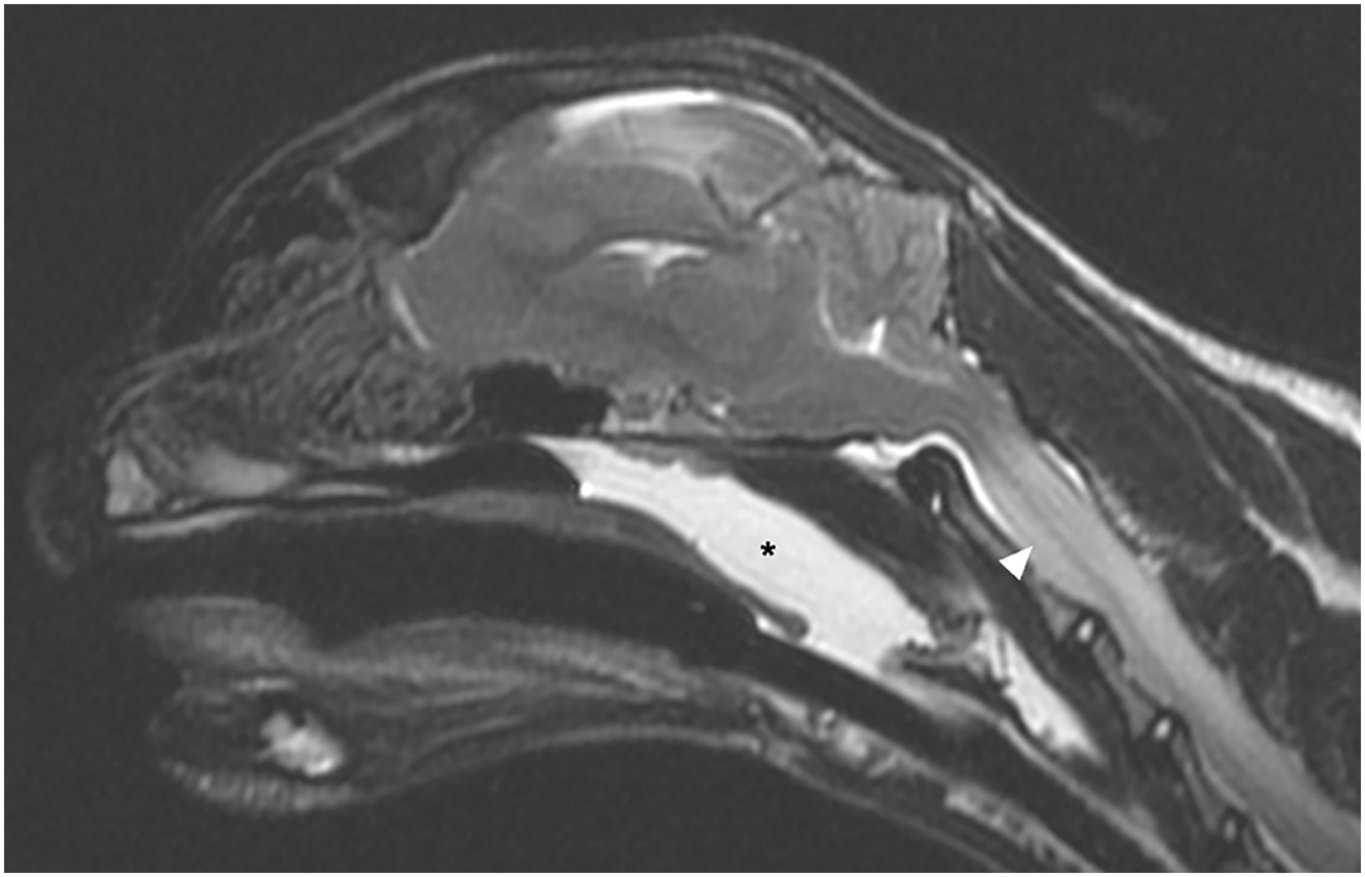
Mid-sagittal T2-weighted (T2W) image of the brain. Note the mass effect with subfalcine, as well as caudal transtentorial, and transforaminal herniation. There is a severe cervical spinal cord swelling and parenchymal T2W hyperintensity (arrowhead). The nasopharynx is fluid filled (*)
